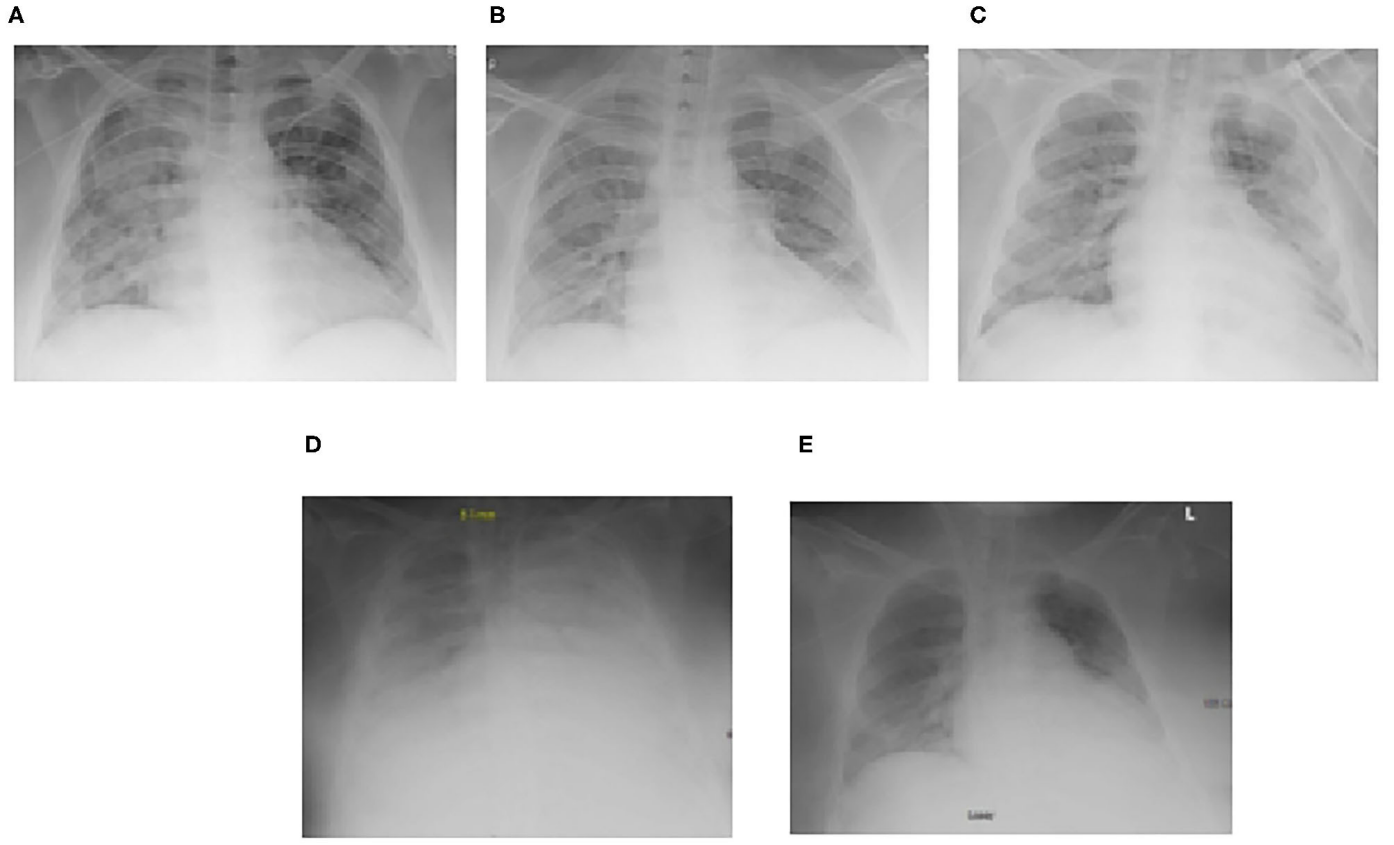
Serial chest radiographs for Case 1 demonstrating the progression and subsequent improvement of diffuse bilateral interstitial and alveolar opacities. (A) Initial decline prompting transfer to the ICU, (B) Mild respiratory improvement, (C) Worsening ARDS leading to transfer for ECMO evaluation, (D) ECMO–Cannulation, (E) Pre-decannulation.
